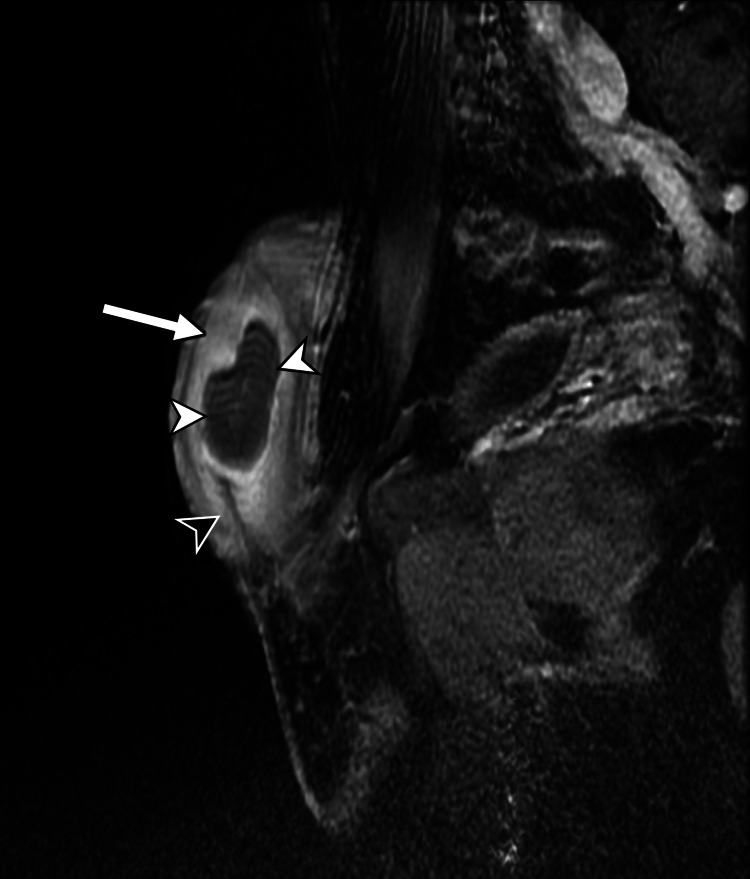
Sagittal fat-suppressed, contrast-enhanced T1-weighted penile MRI The midline haematoma (white arrowhead) is demonstrated centred over the penile septum (black arrowhead). Note how the penile corpora (white arrow) are avidly enhancing, while the central haematoma is not.
